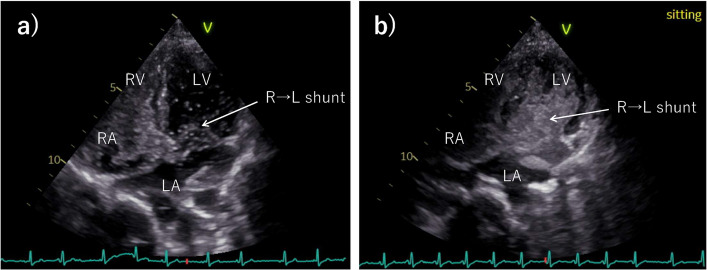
Agitated saline contrast (ASC) transthoracic echocardiographic findings in the supine and sitting positions. In the supine position, the ASC study revealed a small right-to-left shunt, whereas in the sitting position, the shunt was markedly increased. RV, right ventricle; RA, right atrium; LV, left ventricle; LA, left atrium; R → L, right to left
